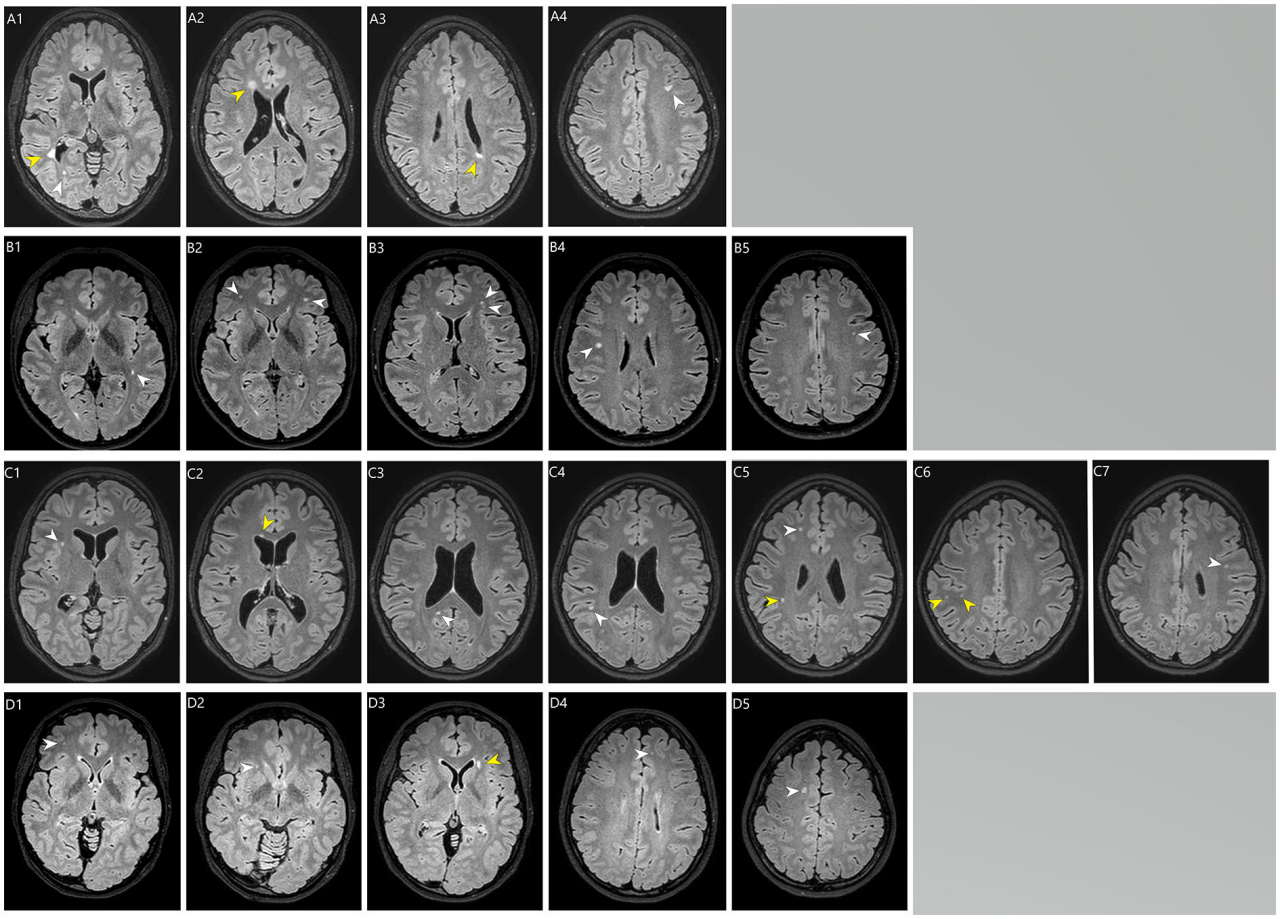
Brain MRI (T2-flair) of the white matter hyperintensities in four cases (Kawasaki disease). (A1–A4) a case (Kawasaki disease) with a total Scheltens' score of 8. (A1) Periventricular WMH > 5 mm in the right occipital horn (yellow arrow; Scheltens' score = 2) and in the deep occipital lobe WMH < 3 mm (white arrow; Scheltens' score = 1). (A2) Periventricular WMH > 5 mm in the right frontal horn (yellow arrow; Scheltens' score 2). (A3) Periventricular WMH > 5 mm in the left ventricle (yellow arrow; no score, because already score has been given from this area). (A4) deep WMH 4–10 mm in the left frontal lobe (white arrow; Scheltens' = score 3). (B1–B4) a case (Kawasaki disease) with a total Schelten score of 6. (B1) deep WMH 4–10 mm in the left temporal lobe (white arrow; Scheltens' score = 3). (B2–B5) multiple deep WMH in the both frontal lobes (white arrows), in the (B2) (left white arrow) and (B4) (white arrow) the size of the largest WMHs are 4–10 mm (Scheltens' score = 3). (C1–C7) a case (Kawasaki disease) with a total Schelten score of 6. (C1,C5,C7) multiple deep WMH < 3 mm in the frontal lobes (white arrows; Scheltens' score = 1). (C2) periventricular WMH ≤ 5 mm in the right frontal horn (yellow arrow; Scheltens' score = 1). (C3) deep WMH < 3mm in the right occipital lobe (white arrow; Scheltens' score = 1). (C4) (white arrow), (C5) (yellow arrow) and (C6) (yellow arrows): deep WMHs in the right parietal lobe, in the (C4) (white arrow) size of WMH is 4–10 mm (Scheltens' score = 1). (D1–D5) a case (Kawasaki disease) with a total Schelten score of 5. (D1,D2,D4,D5) Deep WMHs in the frontal lobes (white arrows). In the (D5) (white arrow) the size of the WMH is 4–10 mm (Scheltens' score = 3). (D3) periventricular WMH size > 5 mm next to left frontal horn (yellow arrow; Scheltens' score = 2).
